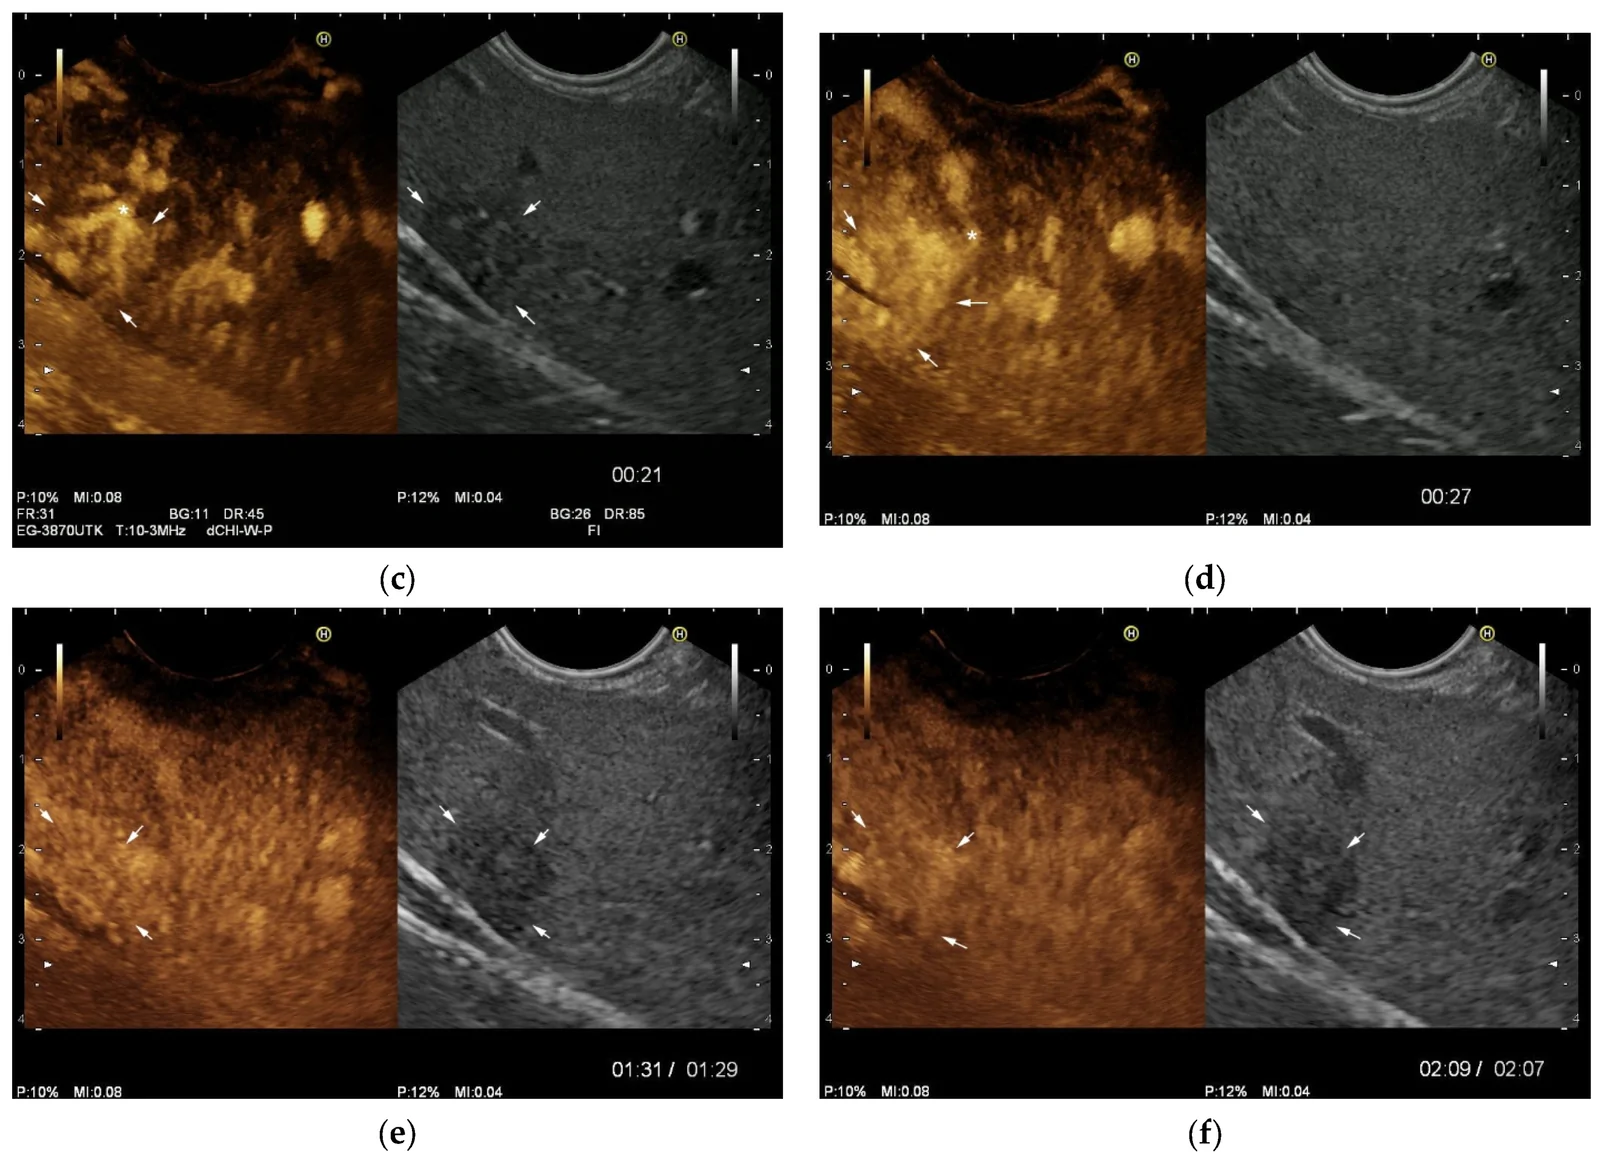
Focal Nodular Hyperplasia. Multiparametric EUS characterisation of a hypoechoic solid FLL with a diameter of 12 × 9 mm found on endoscopic ultrasound in a patient examined for staging of non-small cell lung cancer: B-Mode and Colour Doppler showing a branching vessel entering the lesion ((a), feeding and draining vessels marked with centered arrow and *). Strain elastography demonstrating equal stiffness of the lesion compared to surrounding liver tissue with a strain ratio of 0.97 (b). CE-EUS demonstrating blood inflow from a peripherally located branching vessel into the lesion in early arterial phase ((c); vessel marked with *), diffuse hyperenhancement of the lesion (marked with arrows) only 6 s later in the later arterial phase (d) and persisting hyperenhancement also in the late phase 90 and 129 s post injection of the ultrasound contrast agent SonoVue^®^ (Bracco, Konstanz, Germany) (e,f). The sustained enhancement pattern strongly supported a benign hepatocellular lesion and was considered characteristic of focal nodular hyperplasia in the clinical context.
